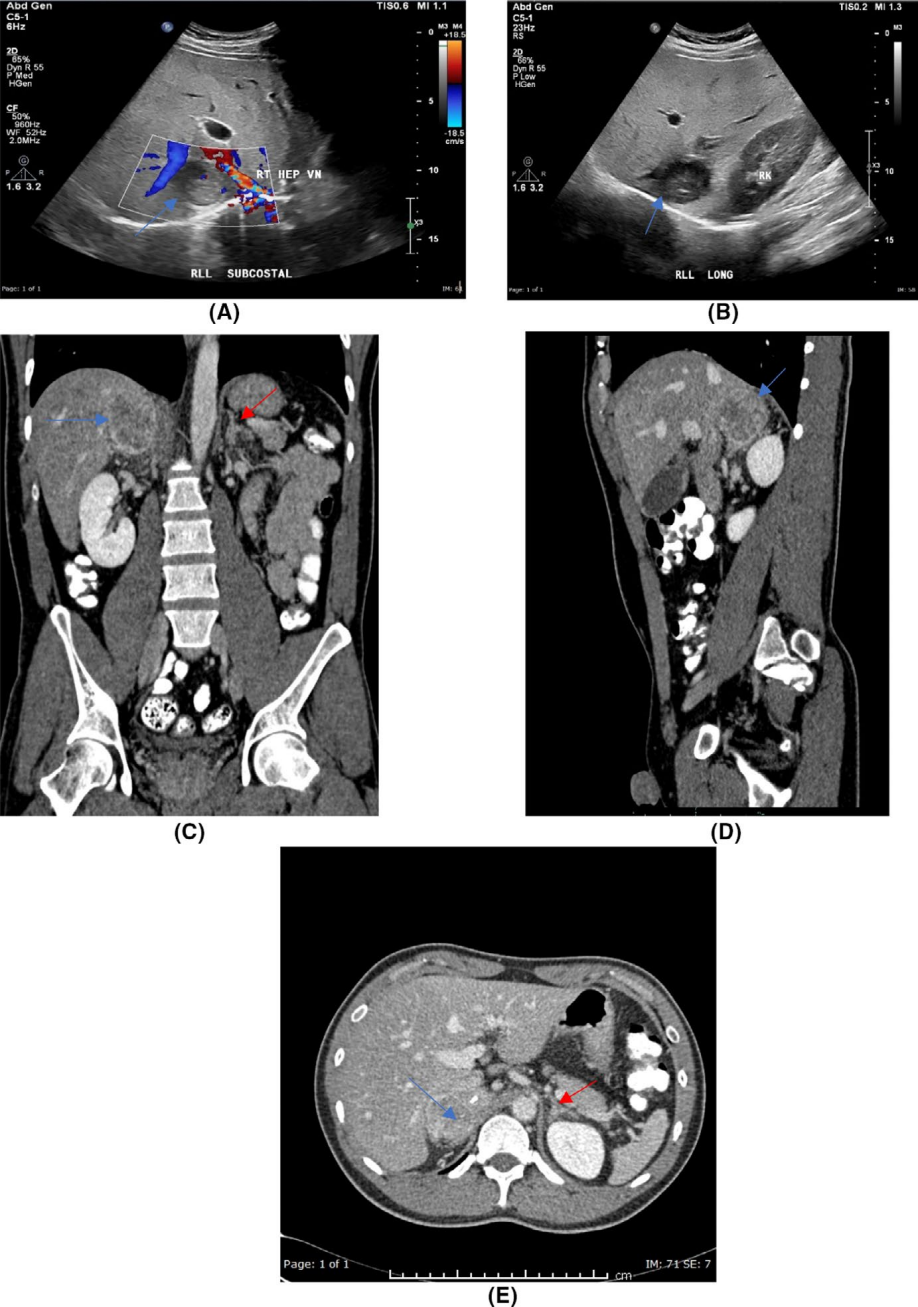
Radiology images (A, B) Abdominal ultrasonography showing focal hypoechoic lesion with mild vascularity by color Doppler related to superior pole of the right kidney and inseparable from right lobe of the liver (Blue Arrows) (C–E) Coronal, sagittal, and axial post contrast computed tomography image of the abdomen showing heterogeneous mass measuring 38 × 34 × 46 mm in the right supra renal gland with central areas of necrosis and calcification inside (blue arrows). The left supra renal gland in normal (Red Arrow in C, E)
